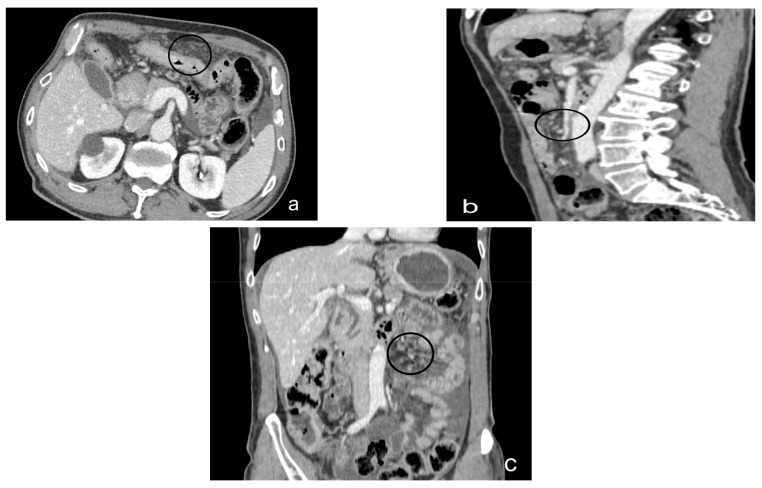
Contrast-enhanced CT scan, axial view (a), sagittal view (b), and coronal view (c). Case of micronodular peritoneal carcinomatosis (black circle), in a male, 75 y, affected by cardias’ adenocarcinoma T4. Diffuse peritoneal involvement with micronodular lesions and ascitis.
